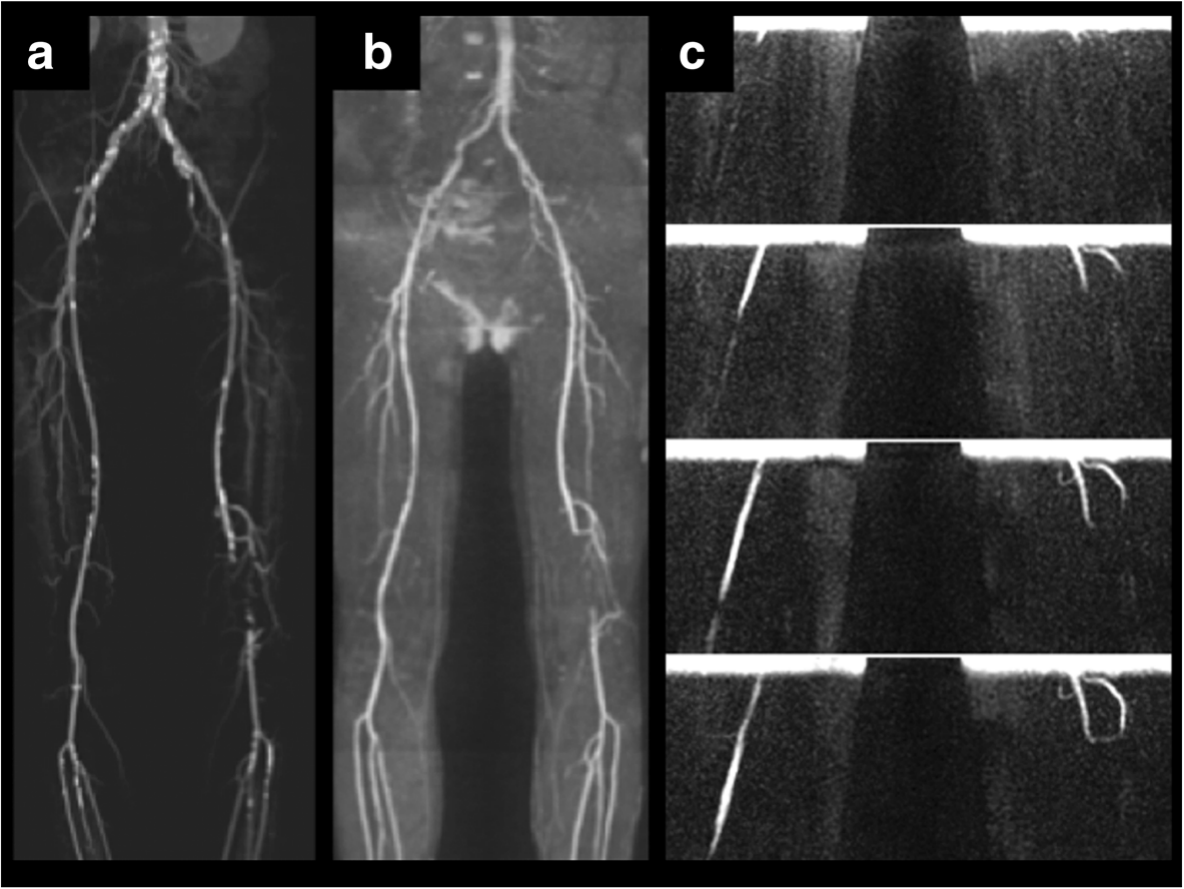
67-year-old male with history of left leg pain. CTA (a) and QISS MRA (b) show occlusion of the distal left superficial femoral artery (SFA) with distal reconstitution through collateral vessels. c Coronal cFASL shows normal, rapid progression of the labeled bolus through the right SFA, in contrast to the markedly delayed progression of the labeled bolus through the left-sided collaterals distal to the occlusion
